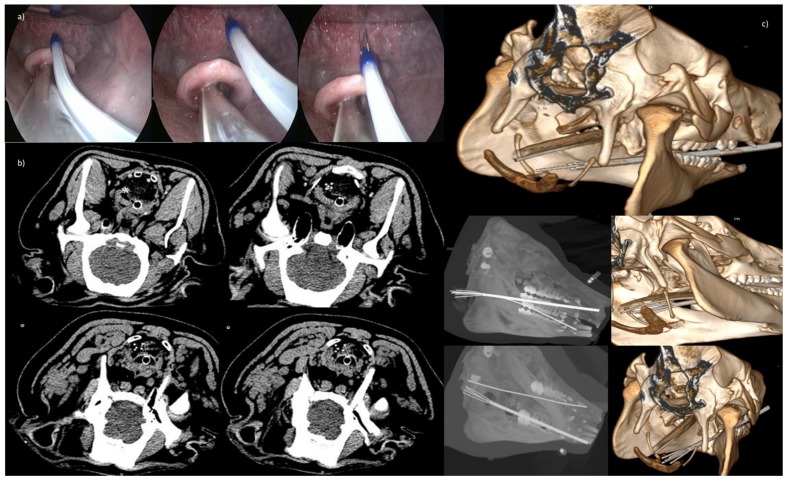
(a) Trans-oral application for intraluminal access to upper airways with the 5-needle expandable electrode (20° of divergence); (b) CT transversal sections in the 3 deployments (2 cm, 3 cm, 4 cm); (c) Volume rendering with central needle exposure of 4 cm and peripheral needles not exposed, and below MIP and Volume rendering, with central needle exposure of 4 cm, and peripheral needle exposure for 3 cm and for 4 cm, respectively.
